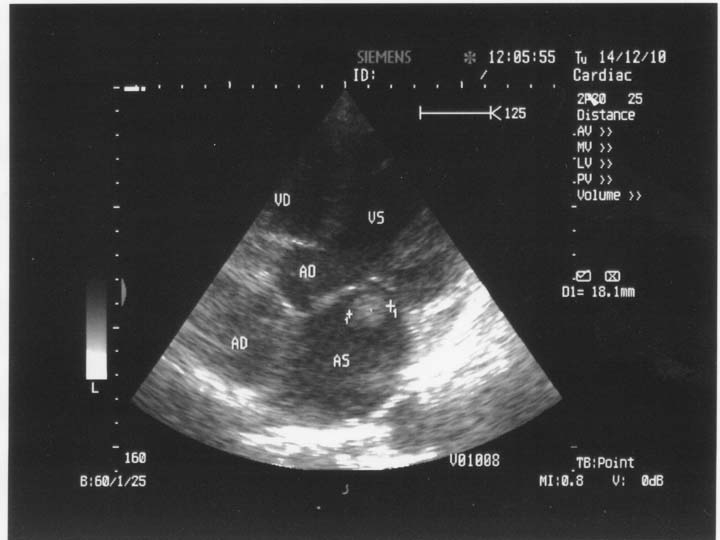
2 D Transthoracic echocardiography. Apical 5 chamber-view. Left intraatrial thrombus between the mitral valves. AS=left atrium, VS=left ventricle, VD=right ventricle, AD=right atrium, Ao=aorta.
